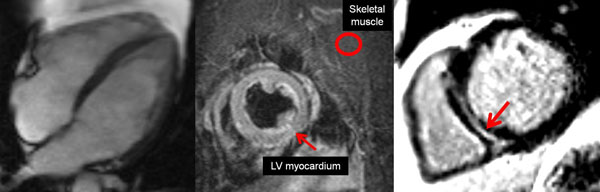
Cardiovascular magnetic resonance imaging techniques for myocardial tissue characterisation. (Left) cine movie imaging for left ventricular function (4-chamber view at end-diastole). (Middle) Oedema imaging using dark-blood T2-weighted CMR. Short-axis image of the ventricles. Note LV myocardial signal intensity compared to skeletal muscle signal intensity is >1.9, consistent with global myocardial oedema. (Right) Late gadolinium enhancement (LGE) imaging. Normal myocardium is black. Red arrow points to a mid-wall scar in the inter-ventricular septum, typical of non-ischaemic type scarring.
